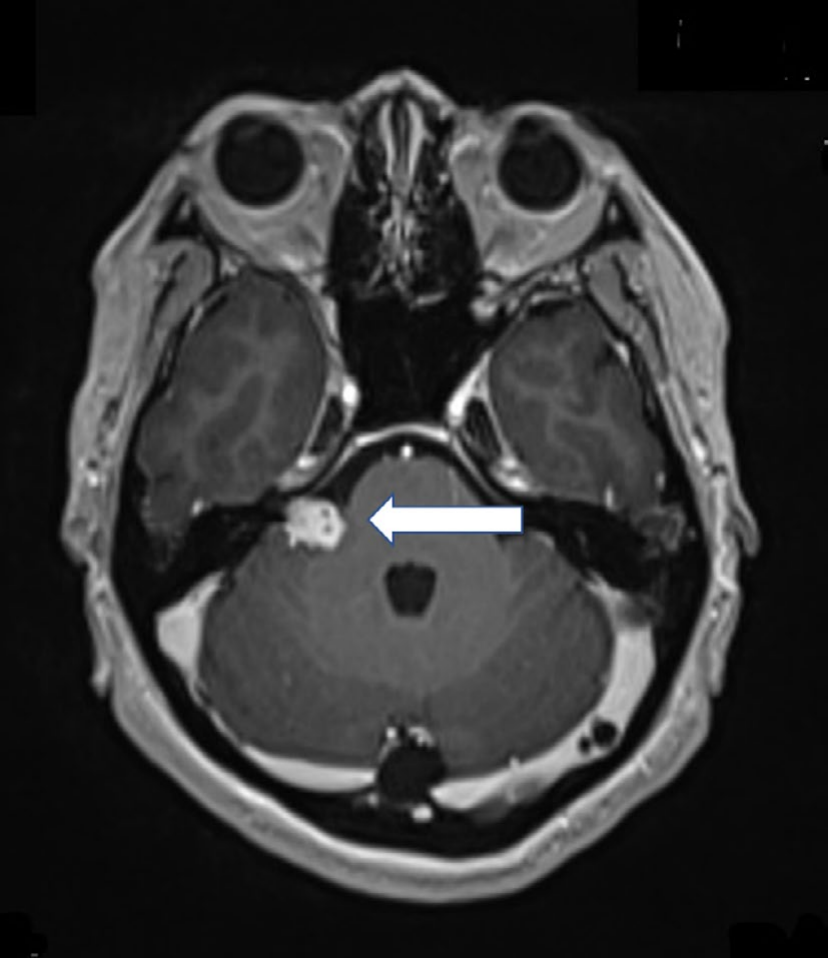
Preoperative brain magnetic resonance imaging. Arrow shows a vestibular schwannoma (16 × 13 mm) at the cerebellopontine angle
